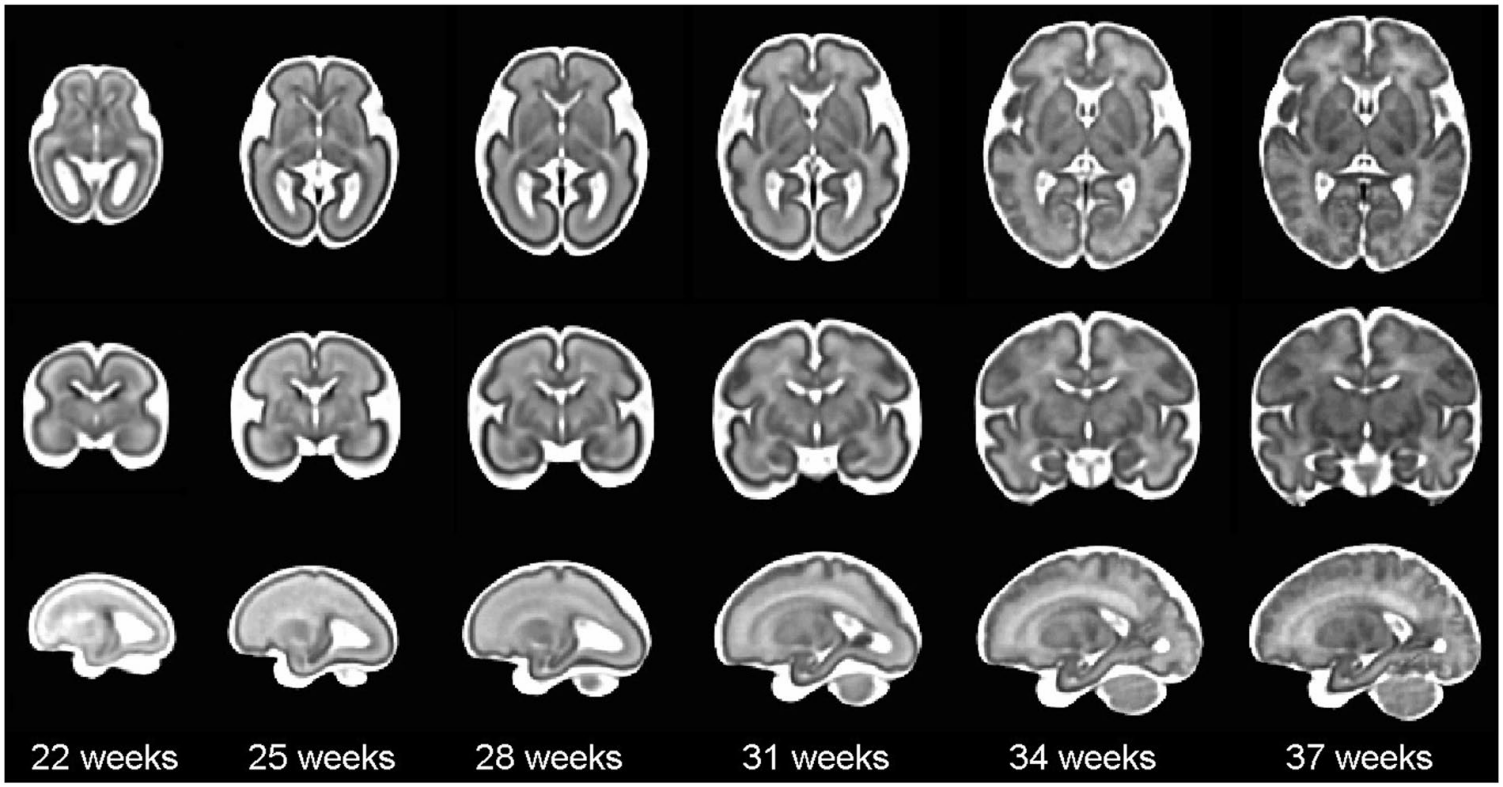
The spatiotemporal fetal brain MRI atlas (CRL fetal brain atlas) at six representative GAs: 22, 25, 28, 31, 34, and 37 weeks. Axial, coronal, and sagittal views of the atlas have been shown at each age point. Note that the spatiotemporal atlas construction process is a time-continuous process, therefore the atlas can be constructed at any continuous age point within the age range of the subjects used in the atlas construction process.
